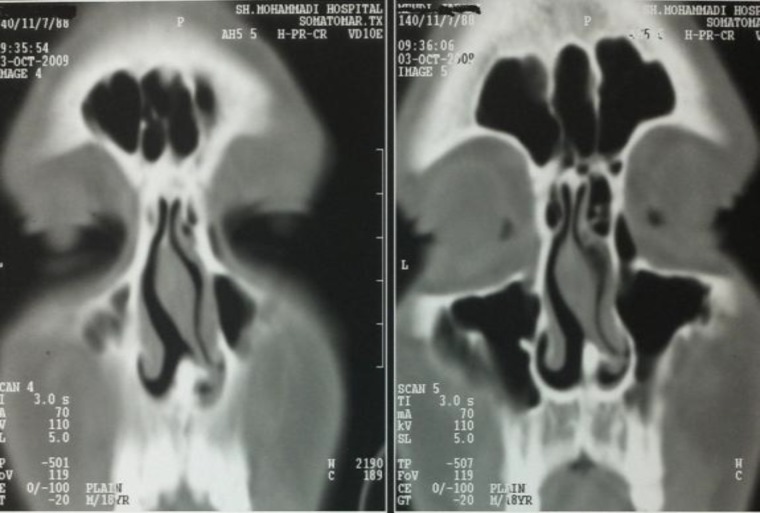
Preoperative CT scan of paranasal sinus.show a bright object in floor of nasal cavity in septum,also severe septal deviation
